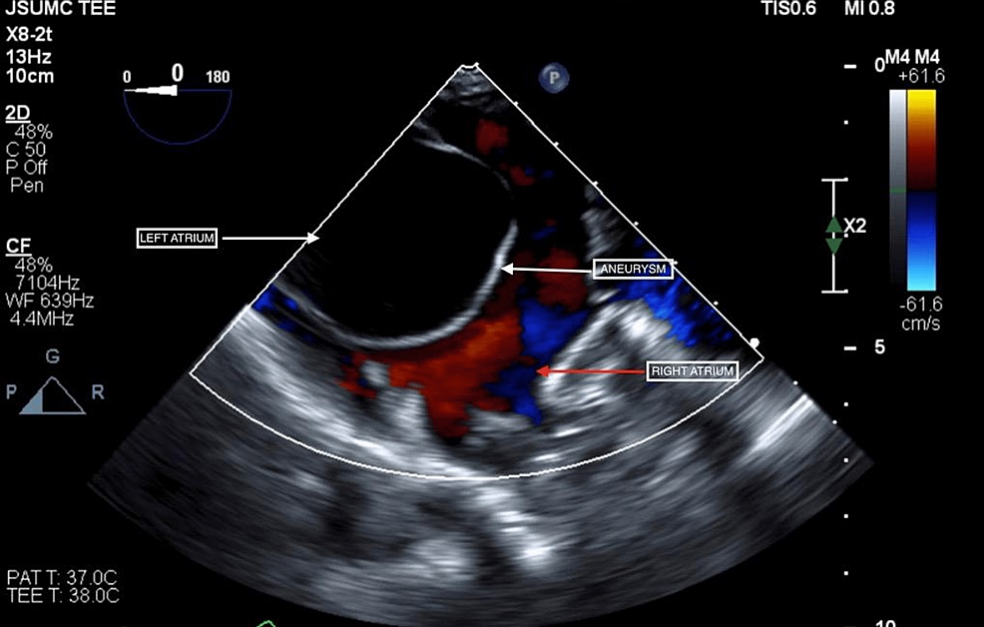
Transesophageal echocardiography (TEE) demonstrating an aneurysmal interatrial septum with fixed left to right bowing
